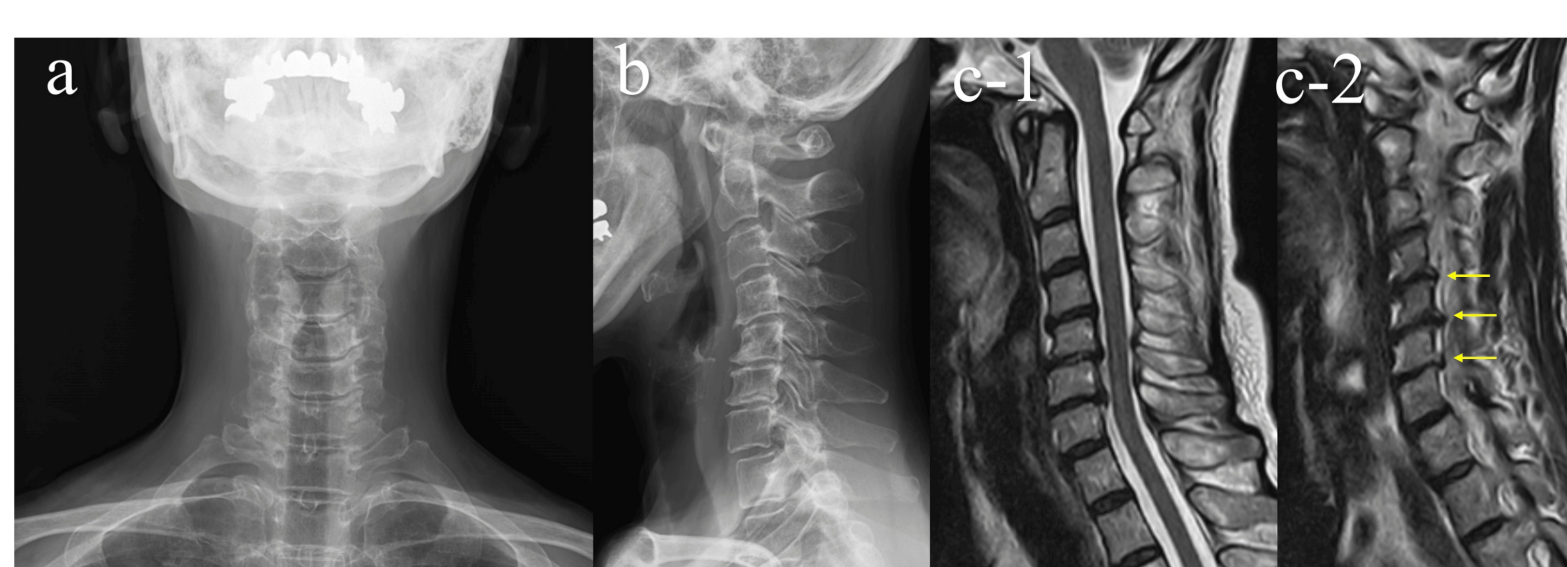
Cervical radiographs and MRI findings. (a) Anteroposterior radiograph showing a sloping-shoulder body habitus. (b) Lateral radiograph demonstrating localized cervical kyphosis. (c-1, c-2) Sagittal T2-weighted MRI showing facet joint osteoarthritis (yellow arrows) and disc bulging at C4/5, C5/6, and C6/7, without evidence of central canal stenosis. MRI, magnetic resonance imaging.
